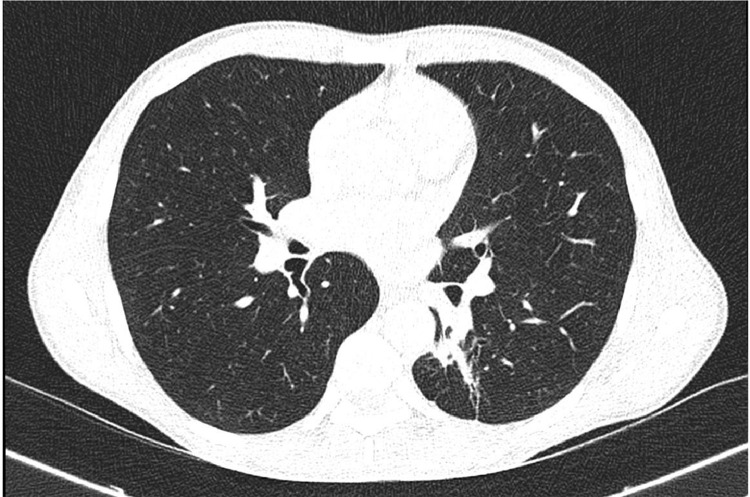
Chest CT done a year and six months later shows complete resolution of the aneurysm and consolidation with mild residual fibrotic changes seen in the superior and posterior segments of the left lower lobe.
